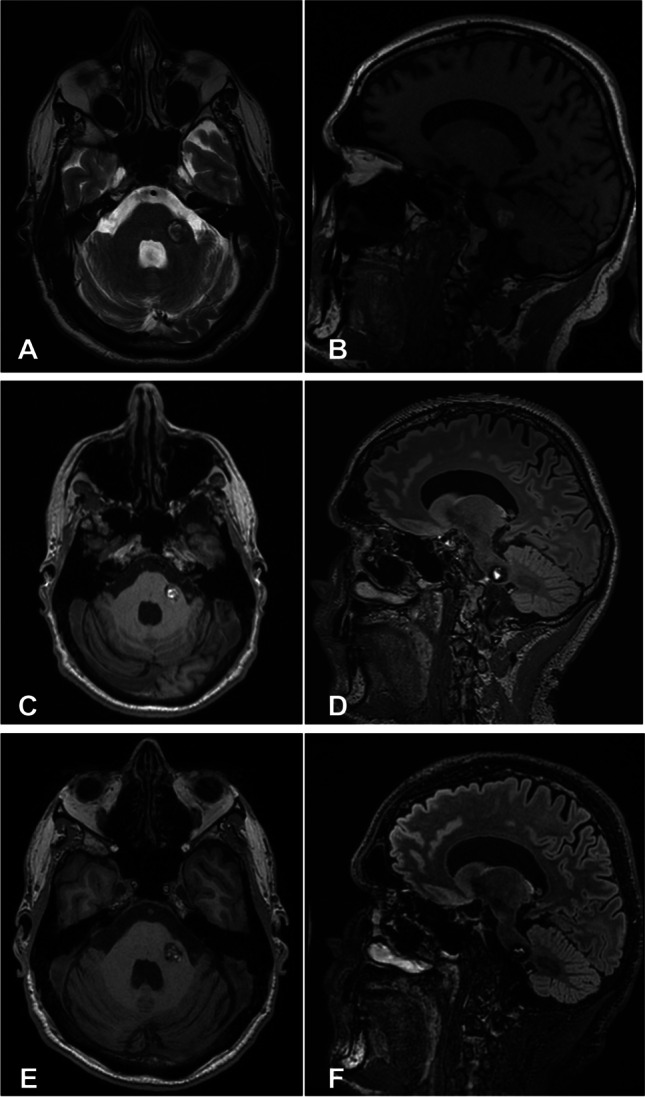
A 41-year-old man presented with repetitive hypesthesia in the anterior left tongue. MRI scan confirmed a 16 × 17 × 13 mm BSCM in the pontocerebellar (A and B). The BSCM score was grade V (size = 1, mRS = 1, hemorrhage event = 1, age = 1, and no midline crossing = 1), and conservative management was recommended. After 2 months, a follow-up MRI scan showed no further progression or fresh bleeding (C and D). The operation score was unchanged, and we recommended the patient for annual follow-up. The last follow-up was 4 years later, with no further deterioration of neurological status (E and F). The BSCM score remained at grade V
